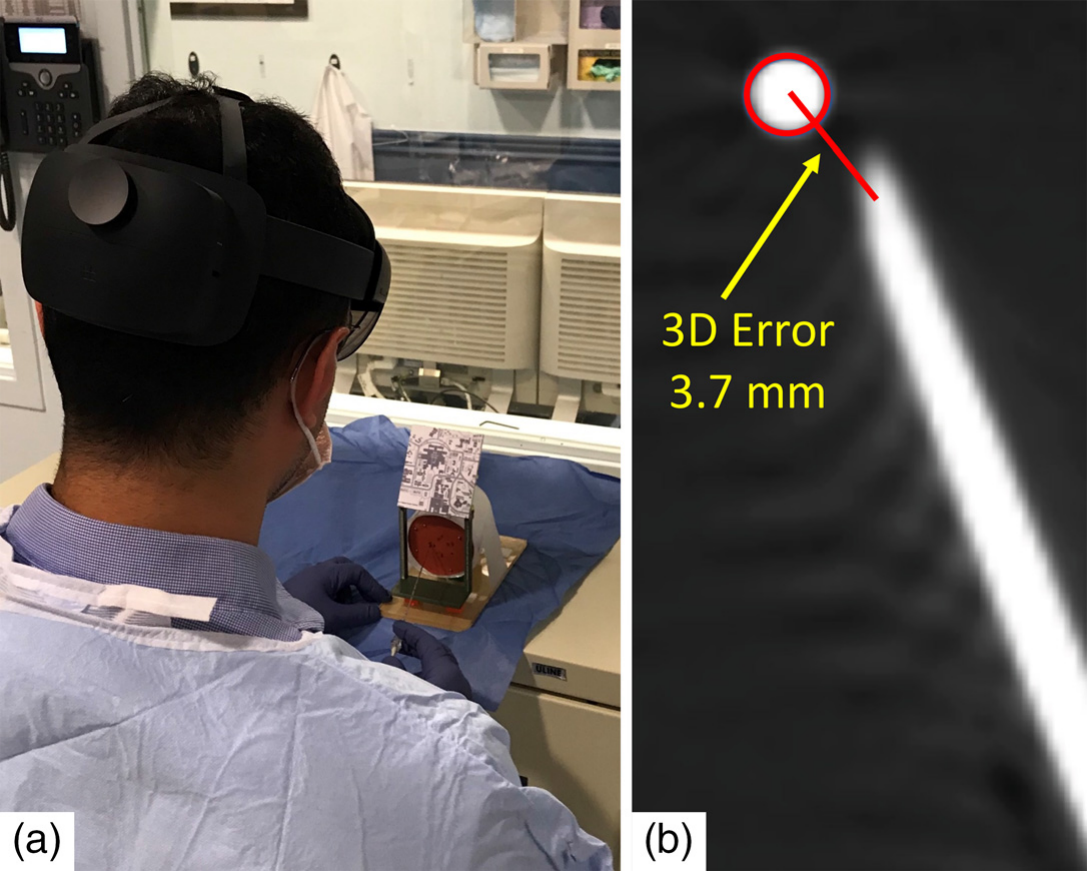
Experiment 2 – performance of HoloLens AR guidance in needle placement task. (a) Scene of a user executing a needle placement task and (b) measurement of the needle placement error was conducted on a postoperative CT. Needle placement error was defined as the distance from the needle tip to the center of the target bead.
